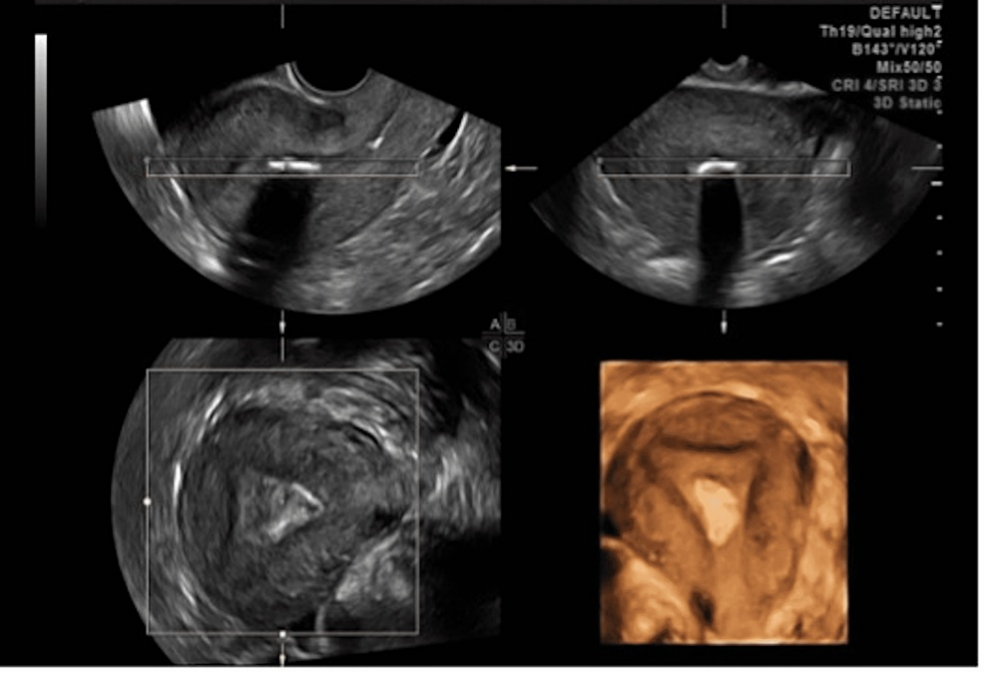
Transvaginal ultrasound image with 3D construction showing the submucosal location of the fibroid
